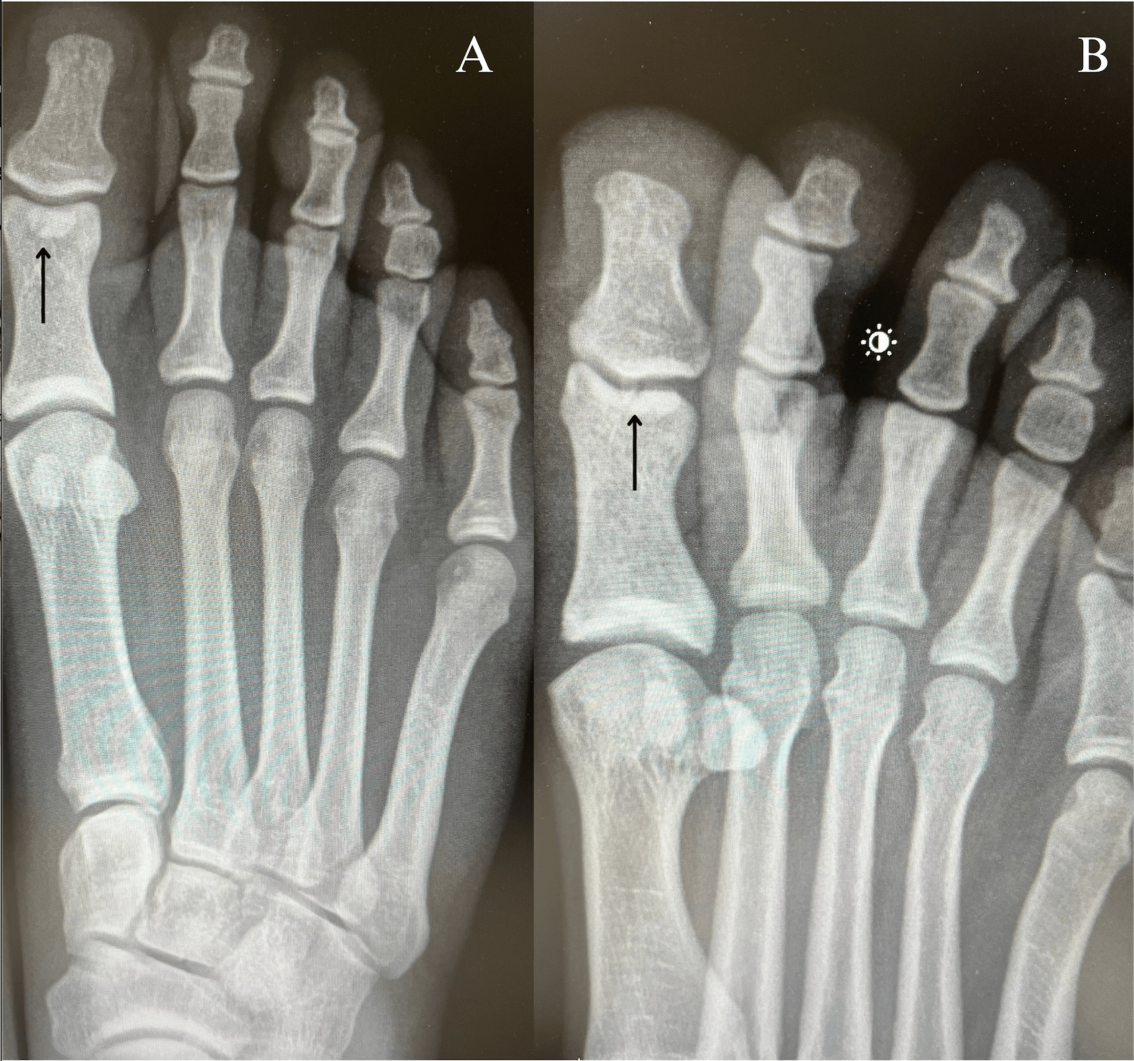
Plain anteroposterior (A) and oblique radiographs (B) of the right foot confirm the presence of a fracture in the interphalangeal sesamoid bone at the plantar aspect of the foot within the distal interphalangeal joint of the great toe (black arrows)
